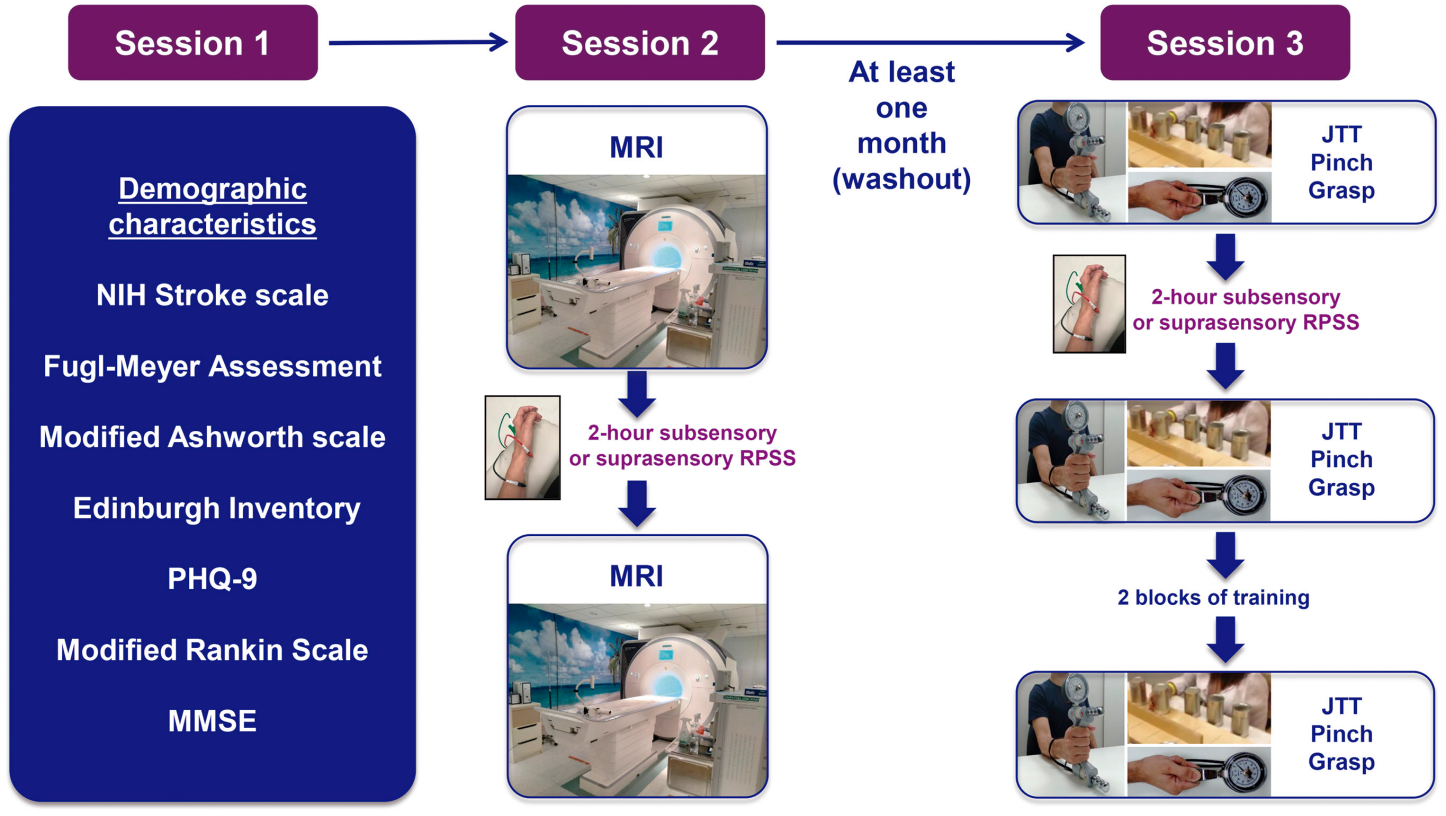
Experimental paradigm. NIH, national institutes of health; PHQ-9, patient health questionnaire-9; MMSE, mini-mental state exam; MRI, magnetic resonance imaging; RPSS, repetitive peripheral nerve sensory stimulation; JTT, Jebsen–Taylor test.
